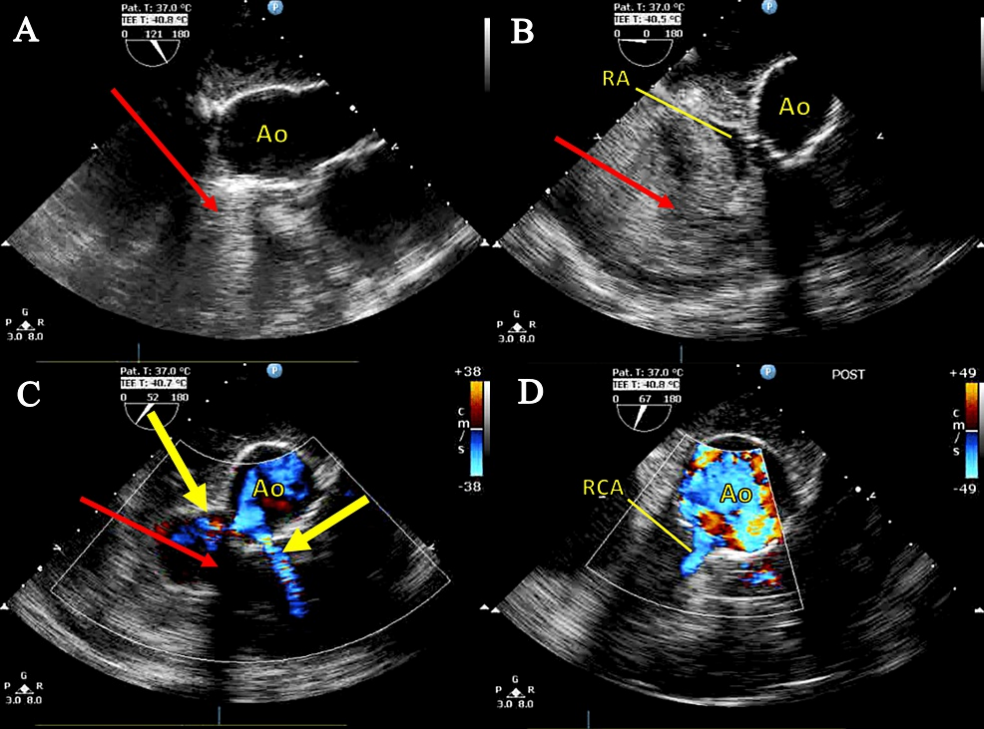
Intraoperative transesophageal echocardiogram images. Pseudoaneurysm with hematoma indicated by a thin red arrow. (A) Mid-esophageal aortic valve long-axis view: right coronary artery (RCA) button pseudoaneurysm anterior to the ascending aorta (Ao). (B) Upper esophageal ascending aortic short-axis view: pseudoaneurysm with compression of the right atrium (RA). (C) Color flow Doppler: two jets of blood flow (thick yellow arrows) visualized feeding pseudoaneurysm. (D) Color flow Doppler: post-repair of the RCA button and evacuation of the hematoma.
